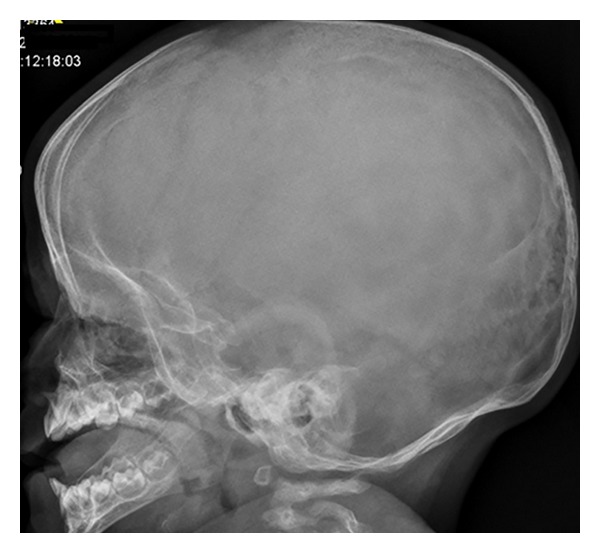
Lateral skull radiograph showed low mineralization of the skull bones associated with persistent opening of the anterior fontanelle. The brain convolutions were apparent because of the demineralized calvaria. Note the bulging frontal area. No evidence of wormian bones and/or dentinogenesis imperfecta.
